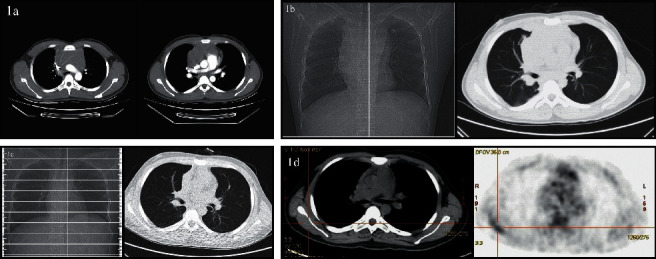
Results of chest CT or PET-CT scan.
